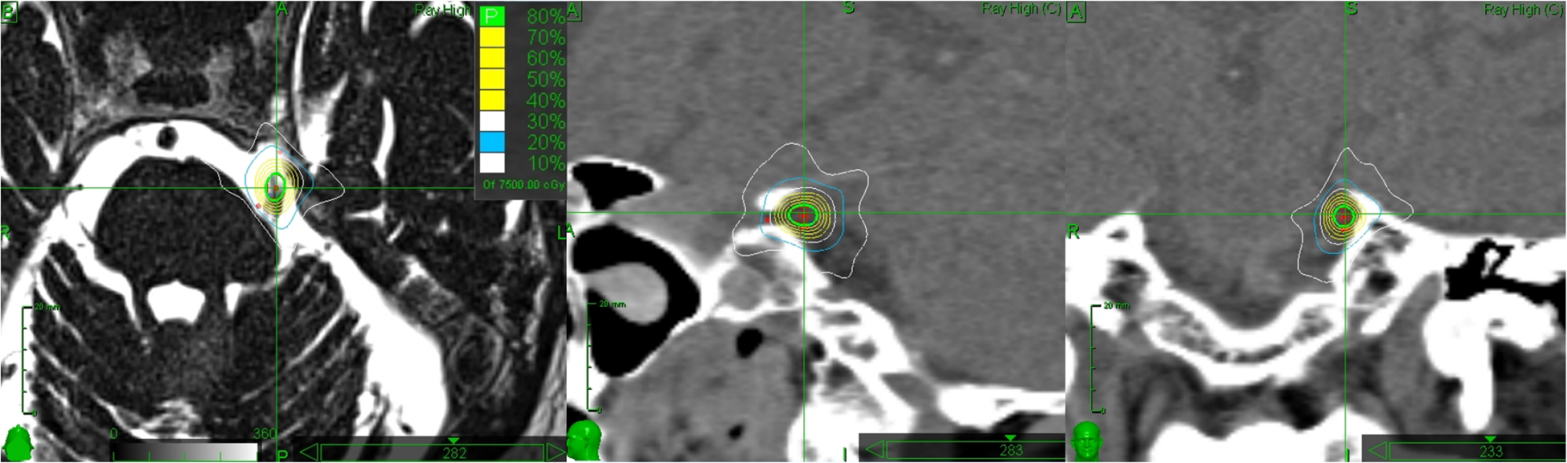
Typical CyberKnife stereotactic dose distribution for trigeminal neuralgia targeted at the cisternal portion of the nerve. left: MR CISS weighted axial image, middle and right: sagittal and coronal CT images. The dose is typically 60 Gy prescribed to the 80% isodose line
